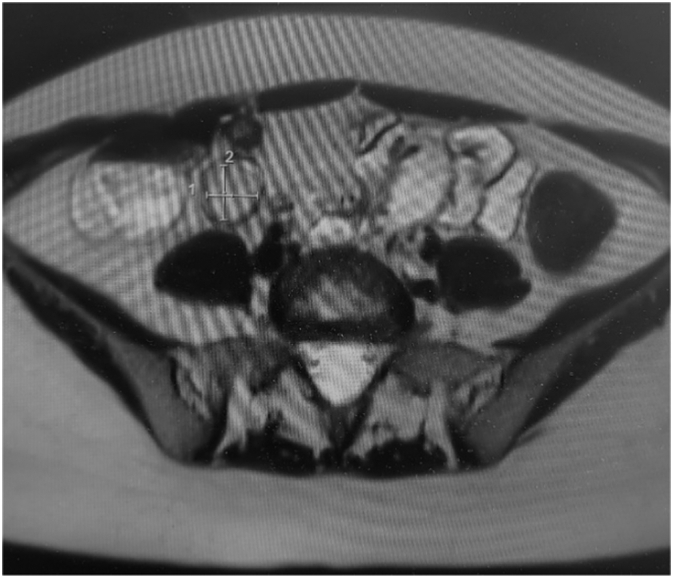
Magnetic Resonance Imaging Enterogram showing heterogenous T2 iso hyperinterse lesion within distal ileum.
